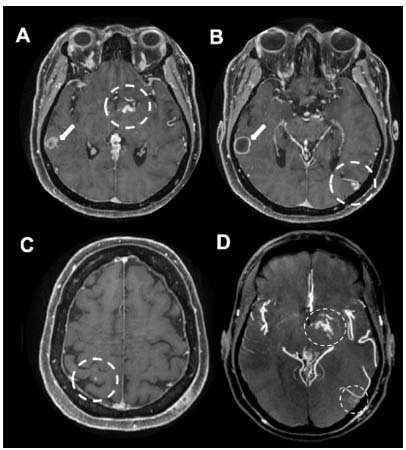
Brain MRI in axial, post-gadolinium volumetric T1 (A to C) and MIP (D) sequences. A pyogenic abscess surrounded by vasogenic edema (arrows) in the right superior temporal gyrus is detected (arrows in A and B). Dashed circles of images A to D highlight multiple tiny vascular malformations in both cerebral hemispheres (capillary malformations and microMAVs), more conspicuous in the MIP sequence.
